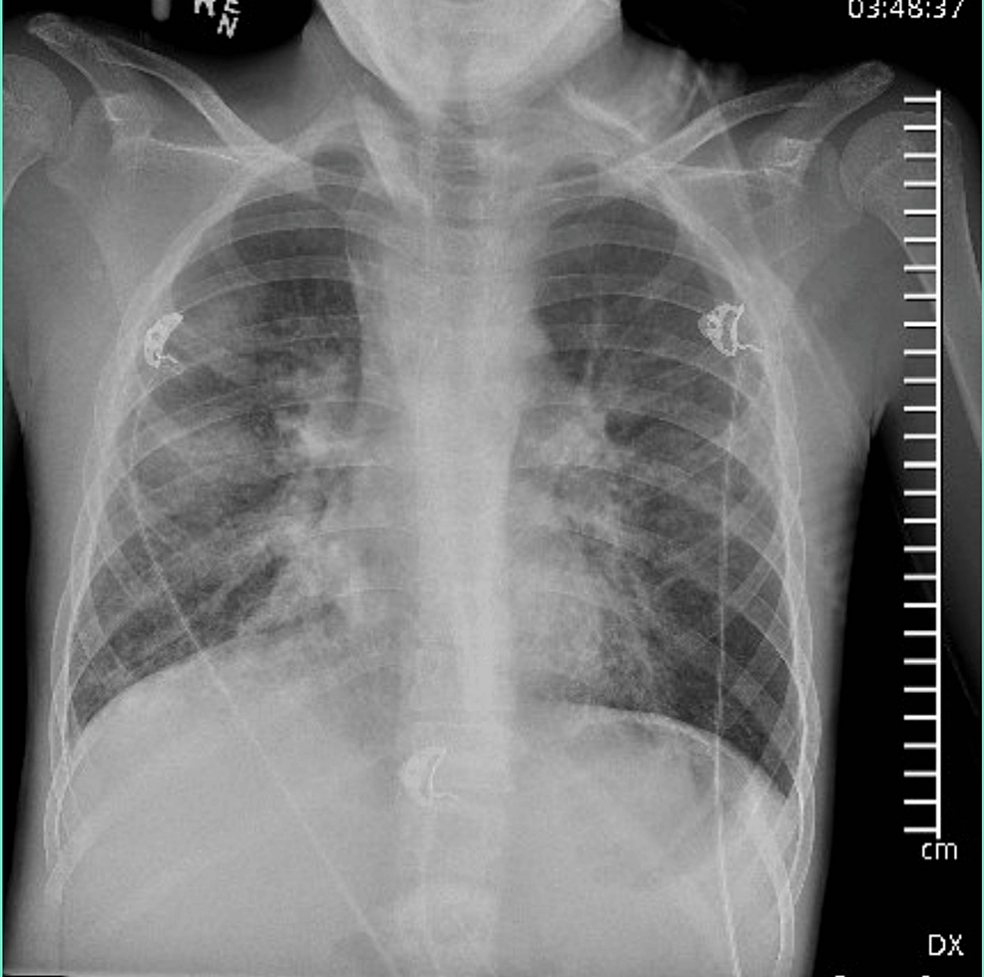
Chest X-ray on initial presentation showing bilateral opacities Chest X-ray showed confluent perihilar opacities as well as bilateral mixed interstitial and airspace opacities, which are highly suspicious for an infectious process.
